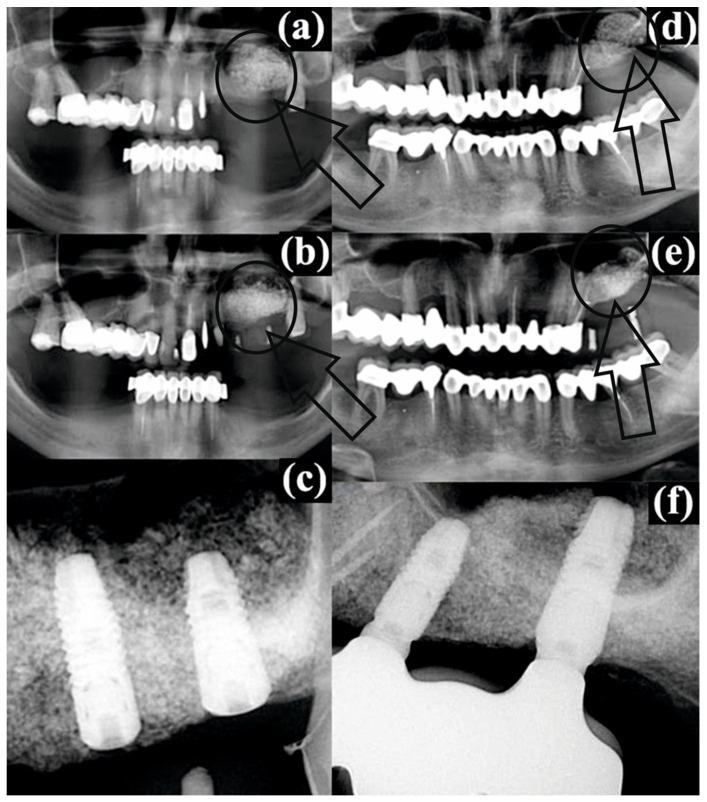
Radiographic images of patient #2: (a) the augmented bone in the left sinus immediately after sinus floor augmentation-highlighted with arrow and circle, (b) postoperative conditions after 4 months of healing-highlighted with arrow and circle, (c) dental implants in the augmented bone at 7 months after augmentation; and patient #3: (d) the augmented bone in the left sinus immediately after sinus floor augmentation-highlighted with arrow and circle, (e) postoperative conditions after 4 months of healing-highlighted with arrow and circle, (f) dental implants in the augmented bone at 7 months after augmentation. The augmentation loci are encircled and arrow are pointing to the regions of interest.
